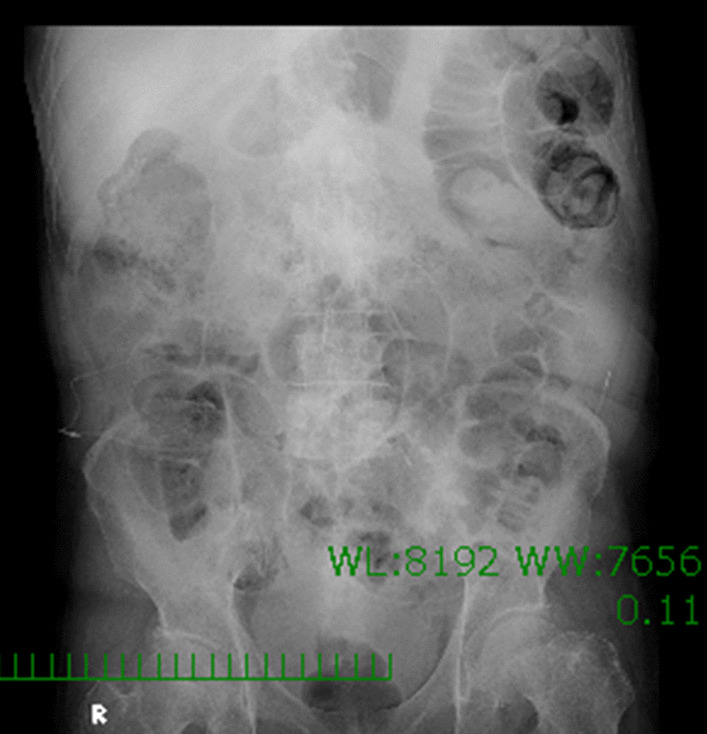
Simple abdominal radiography. Simple abdominal radiography shows gas and stool retention in the intestinal tract; however, intestinal obstruction is negative
